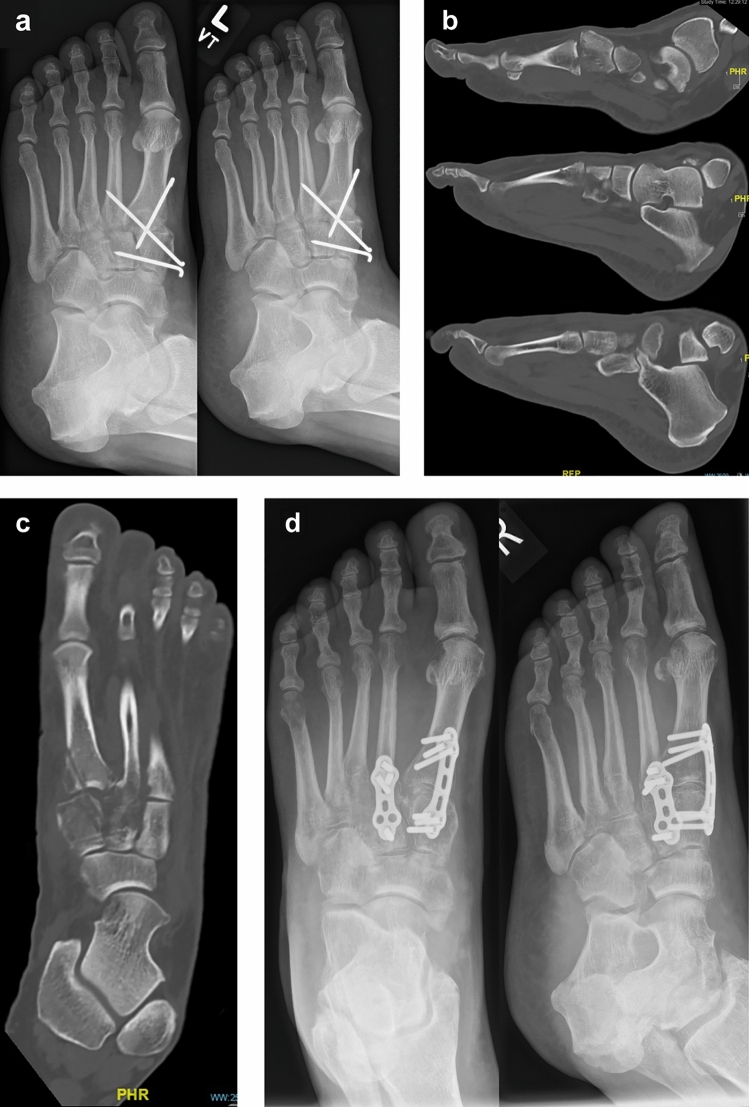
A 39-years-old female patient had a distortion trauma of her right midfoot resulting in a Lisfranc injury involving first and second tarsometatarsal joints. (a) She underwent a closed reduction of the Lisfranc joint and transfixation using Kirschner-wires (in an outside hospital). (b) Eight weeks later Kirschner wires were removed, and CT scan was performed demonstrating substantial dislocation of the first (top) and second (middle) tarsometatarsal joints. The alignment of the third tarsometatarsal joint was OK (bottom). (c) Severe dislocation of the first and second tarsometatarsal joints has been also confirmed using axial CT scan. (d) Revision surgery was performed including open anatomic reduction of both dislocated joints and transfixation using two locking plates.
